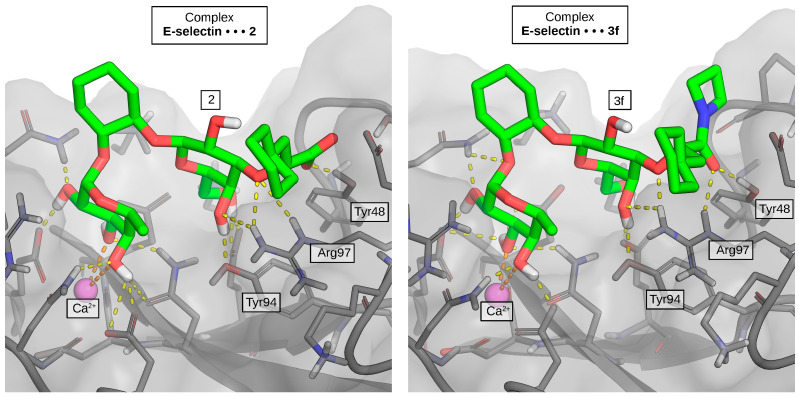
Prepared X-ray structures of the lead structure 2 (left) and the azetidine amide 3f (right) in green sticks representation showing consistency in binding modes (calcium ion is represented by a purple sphere, protein surface semitransparent).
